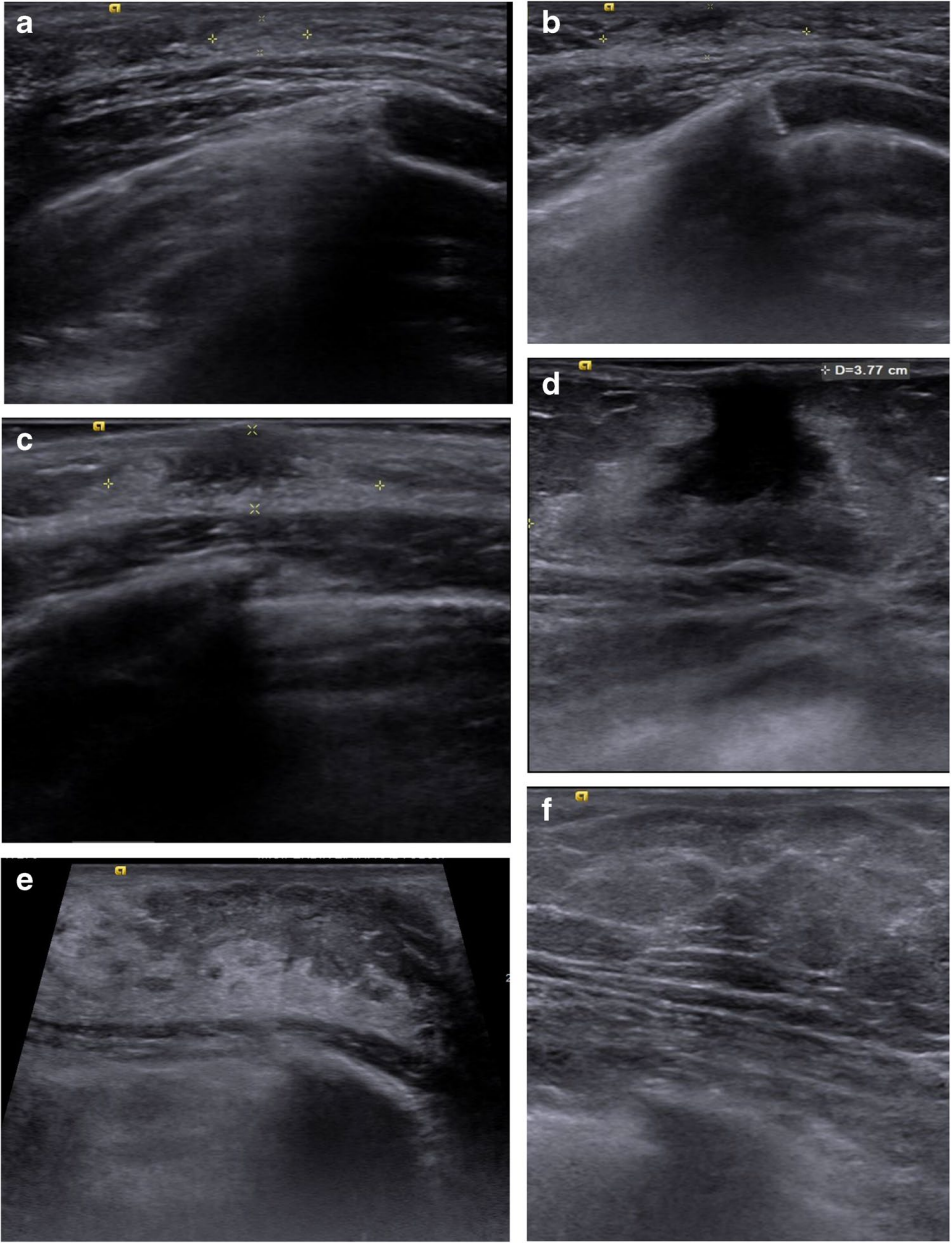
Axial plane grayscale breast ultrasound images show ultrasonographic staging of breast development (a–e) and lipomastia (f). a Stage I in a 6-year-old girl with an ill-defined hyperechoic area in the retroareolar zone (*calipers*). b Stage II in a 7-year-old girl with a hypoechoic core and hyperechoic nodule limited to the retroareolar area (*calipers*). c Stage III in an 8-year-old girl with a spider-shaped hypoechoic nodule within hyperechoic tissue exceeding subareolar area (*calipers*). d Stage IV in an 8.5-year-old girl with significant amount of hyperechoic tissue with secondary branching hypoechoic nodule. e Stage V in a 12-year-old girl with prominent hyperechoic tissue without central nodule. f An 8-year-old girl with prominent retroareolar adipose tissue but without any glandular breast tissue (lipomatous pattern)
